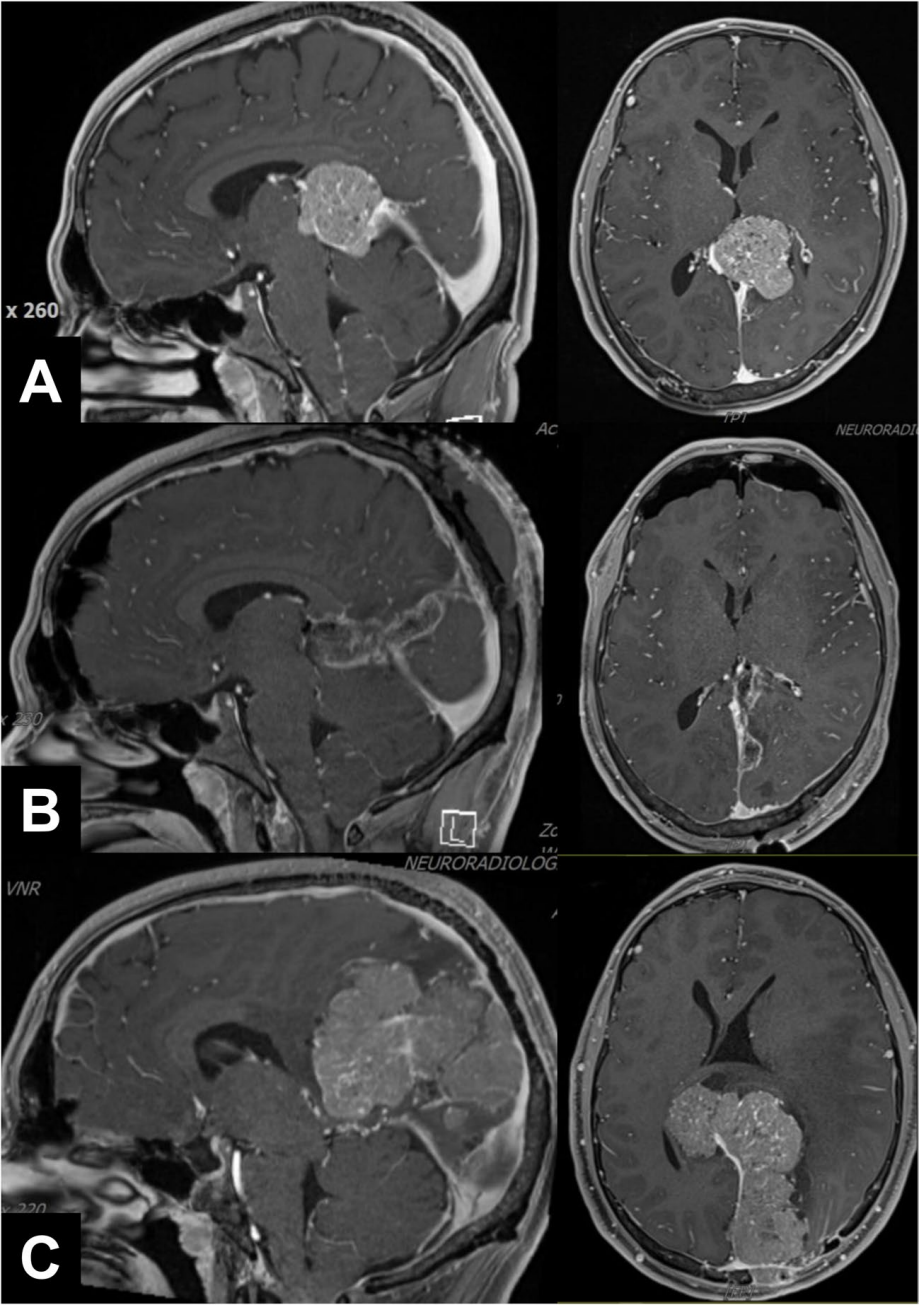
A Atypical falcotentorial meningioma WHO/CNS grade II operated following a first generalized seizure in a 20-year-old male. B Postoperative MR imaging confirmed the intraoperative impression of a Simpson grade III resection. C The patient did not report back to our center until 53 months later. At this time, there was a very large recurrence likely originating from the tumor infiltrated dura left behind during the initial surgery. However, resecting the dural tumor origin, i.e., the sinuses involved in this case during the first operation would not have been safe
